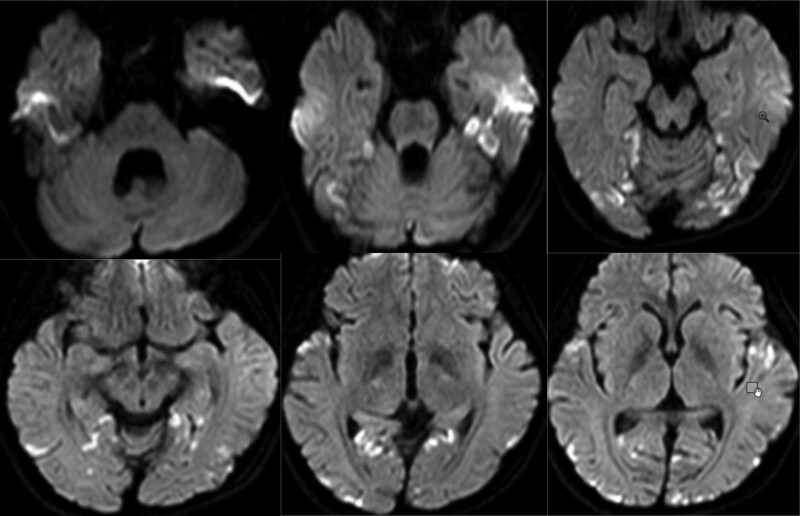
Magnetic resonance imaging presents abnormal signals in bilateral temporal lobes, hippocampi, occipital lobes, and frontoparietal regions, with high signal intensity in the basal ganglia in T1-weighted imaging.
